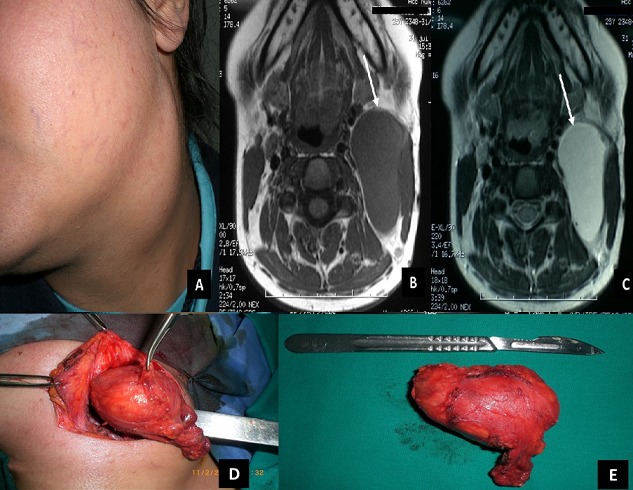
A) clinical image showing a mass of the upper-left neck; B) axial T1-weighted MRI shows a well-defined hypointense mass that was confined to the left aspect of the neck (arrow); C) the mass (arrow) was hyper-intense on T2-weighted sequences (Axial T2-weighted MRI); D) peroperative view; E) the excised mass was an encapsulated cystic structure
